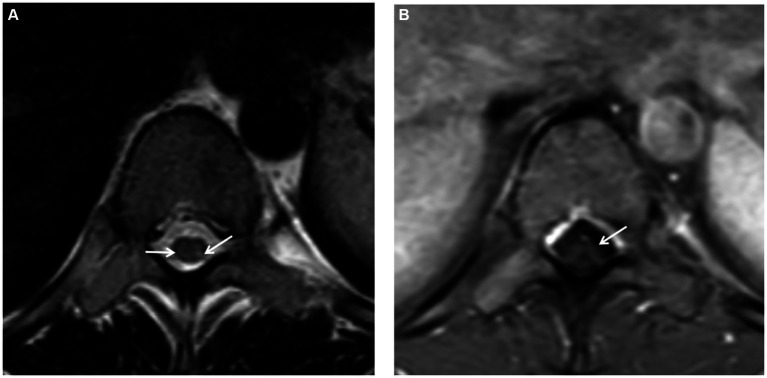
(A) On the T2-weighted imaging (T2WI) sequence of the transverse section, small spot-like signals were detected in the posterior and lateral funiculus of the spinal cord at the T10 level, with slightly more significant presence on the left side. (B) Following gadolinium contrast enhancement scanning on the T1-weighted imaging (T1WI), a “reverse V-shaped” high signal was evident.
